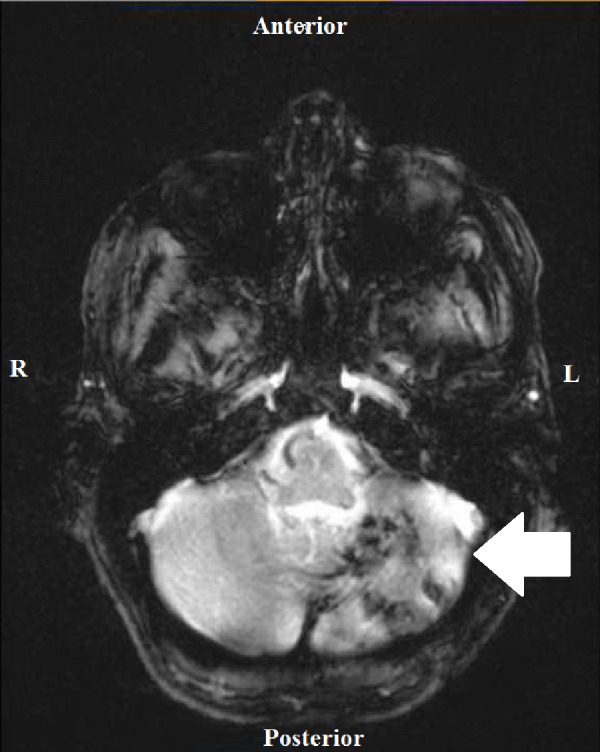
Brain Magnetic Resonance Imaging after Craniotomy Brain MRI (T2 Weighted) without gadolinium completed two months after left occipital craniotomy and evacuation of infarcted left cerebellar tissue. Images demonstrate left cerebellar subacute and chronic infarctions as well as an area of acute infarction within the pons.
